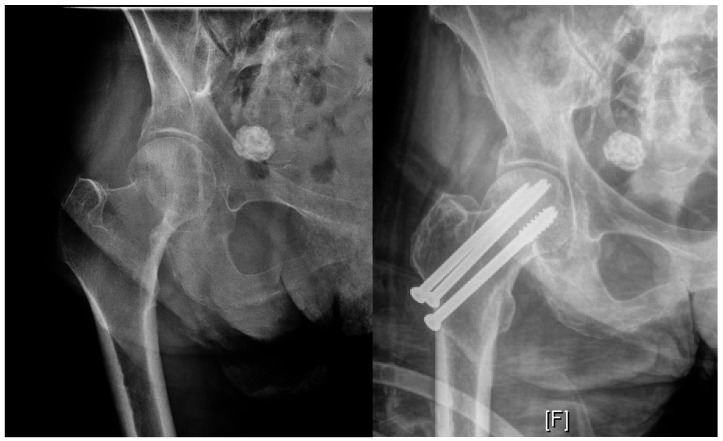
Example radiographs demonstrating internal fixation for Garden I (Left: Preoperative, right: Postoperative).
